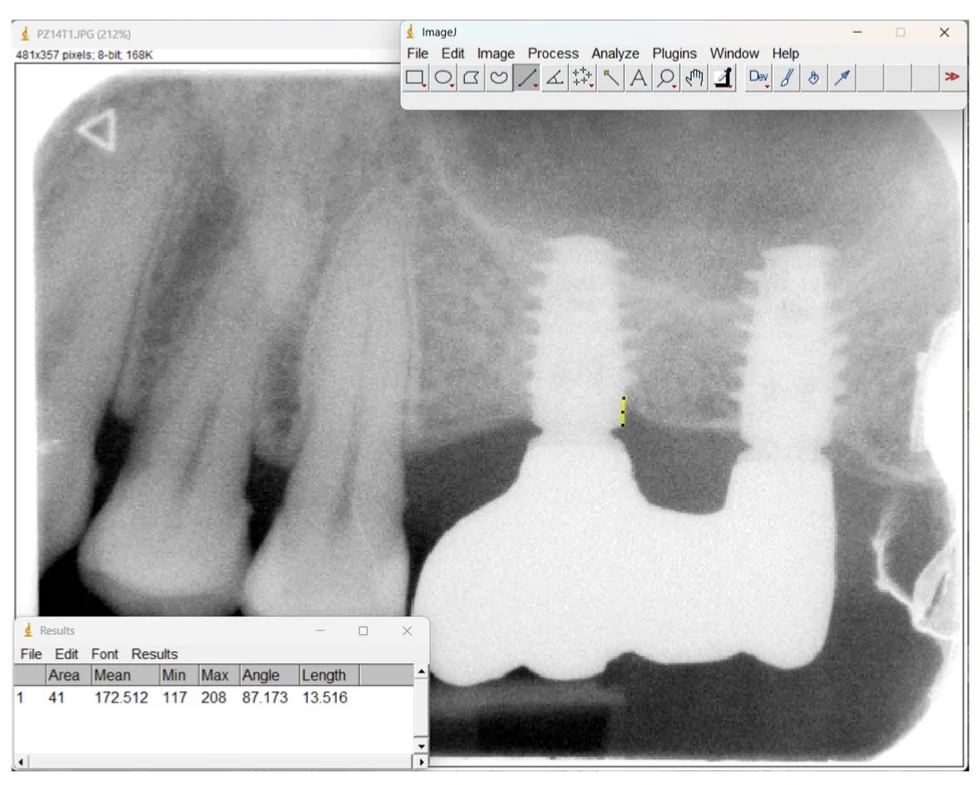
Example of radiographic analysis using ImageJ.
